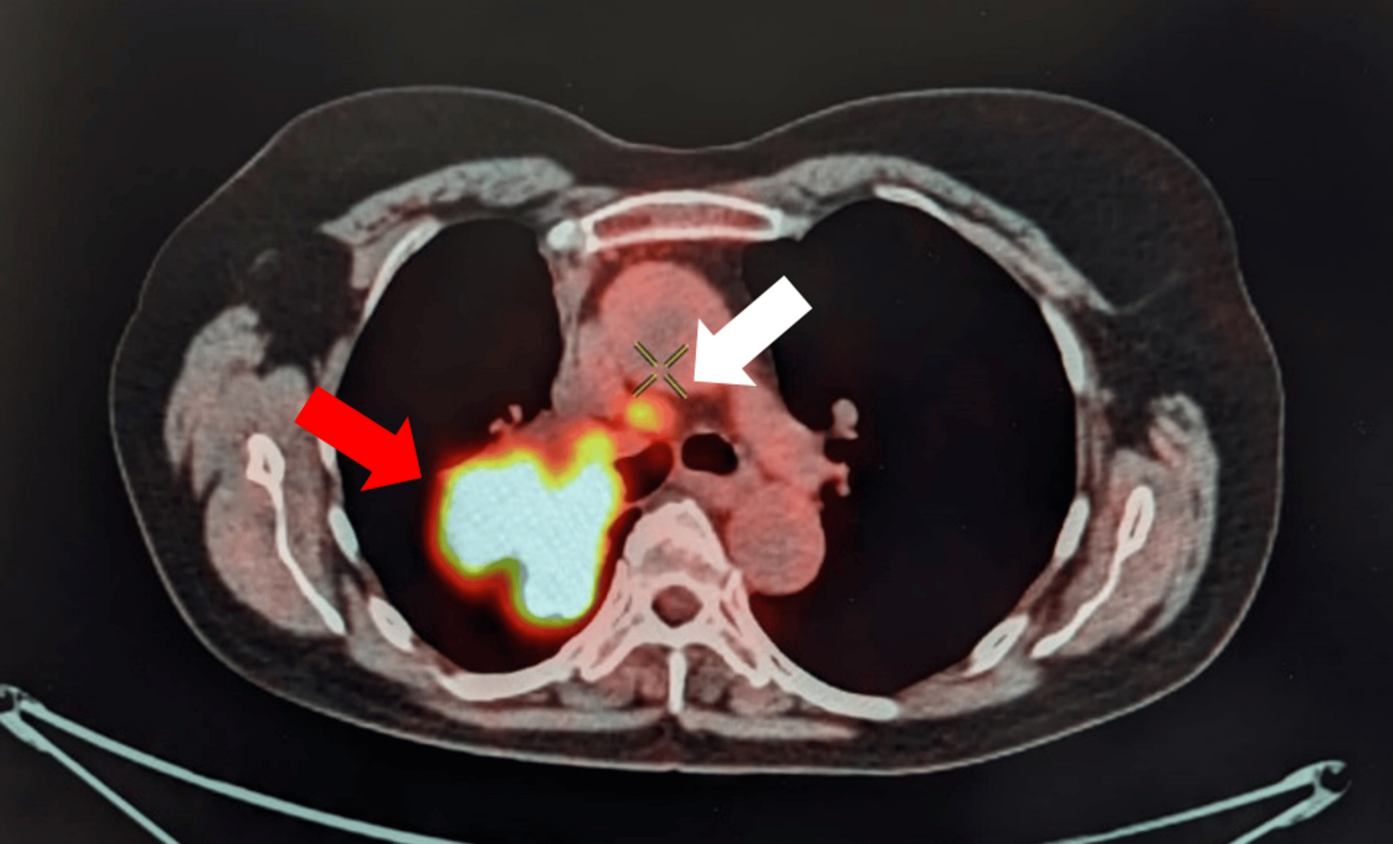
PET-CT image showing a hypermetabolic mass in the right lung (indicated by the red arrow) and a hypermetabolic lower paratracheal lymph node (indicated by the white arrow) PET-CT: positron emission tomography CT
